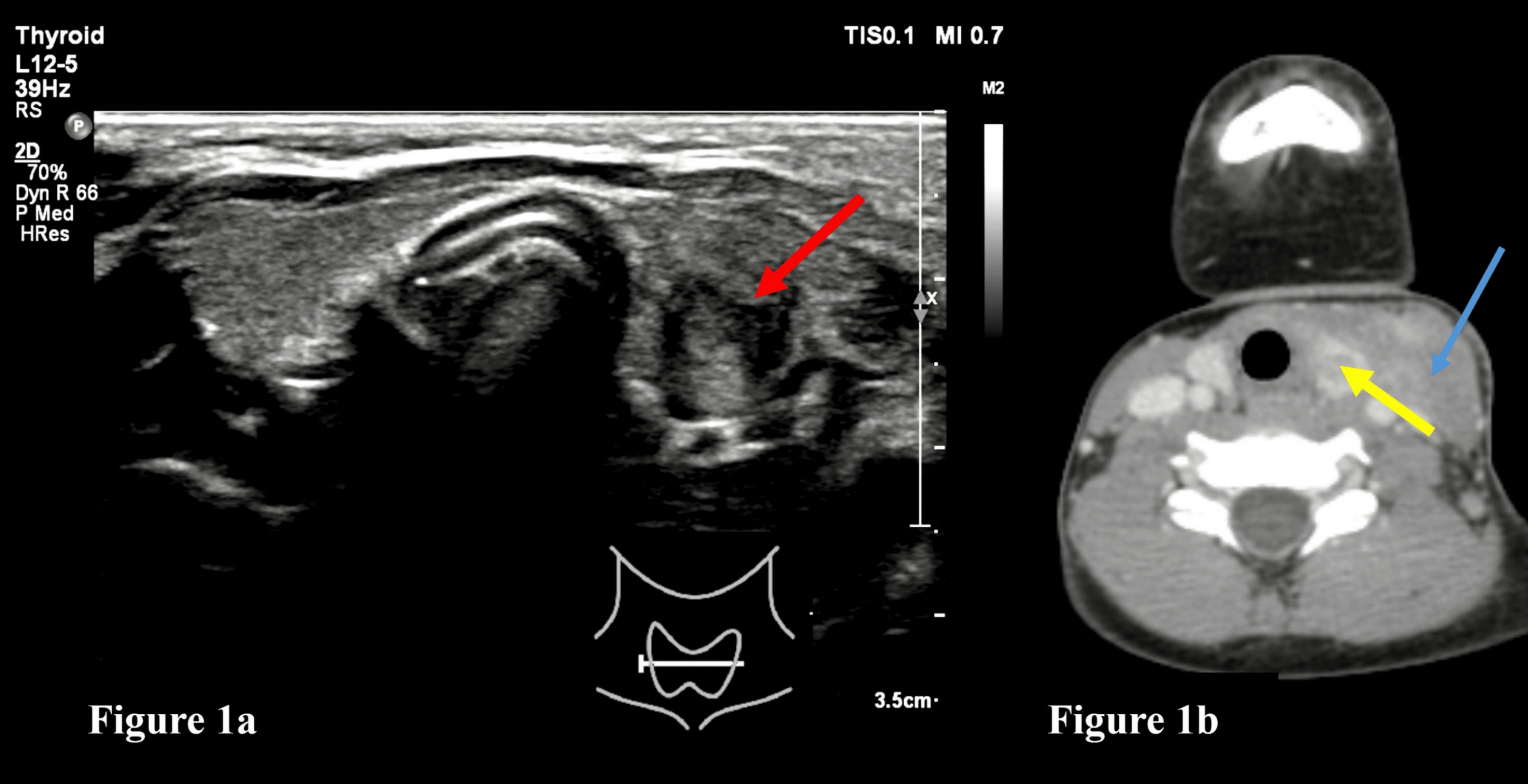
Ultrasound and CECT of the neck a: Ultrasonography of the neck region (transverse section) shows a heterogenous hypoechoic lesion involving the left lobe of the thyroid (red arrow); b: Axial section of contrast-enhanced computed tomography (CECT) of the neck shows a conglomerate lymph nodal mass on the left side of the neck (blue arrow), with extension into the left lobe of the thyroid (yellow arrow).
